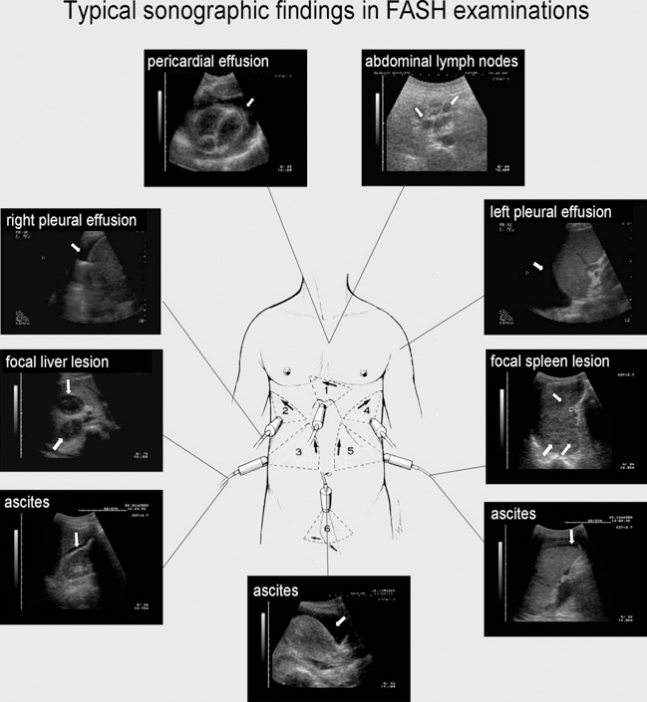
Pictorial summary of the focused assessment with sonography for human immunodeficiency virus/tuberculosis (HIV/TB) (FASH).
